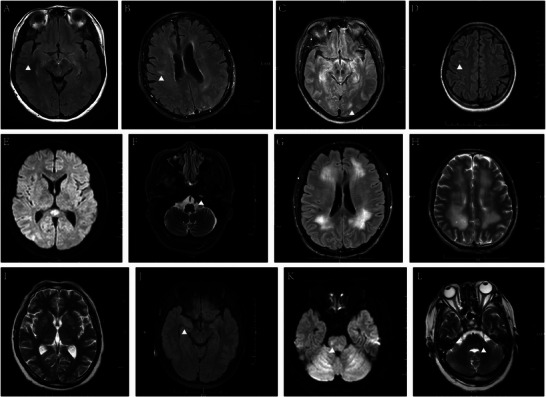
Different manifestations on cranial MRI in patients with GFAP‐A. (A) Swelling of the temporal lobe on the T2 flare sequence, (B) Patchy high signal in the right parietal lobe on the T2 FLAIR sequence, (C) Small nodular high signal in the left occipital lobe on the T2 FLAIR sequence, (D) Punctual abnormal signal in the right frontal lobe on the T2 FLAIR sequence, (E) The DWI sequence shows high signal intensity in the area of corpus callosum compression, (F) High signal intensity at the apex of the left temporal bone on the T2 sequence, (G) High signal intensity near the lateral ventricle on the T2 FLAIR sequence, (H) High signal intensity in the center of the semioval region on the T2 sequence, (I) High signal intensity in the basal ganglia region on the T2 sequence, (J) Hippocampal sclerosis on the T2 FLAIR sequence. (K) High signal intensity on the right side of the brainstem on the DWI sequence, and (L) High signal of the left pontine arm on the T2 sequence.
